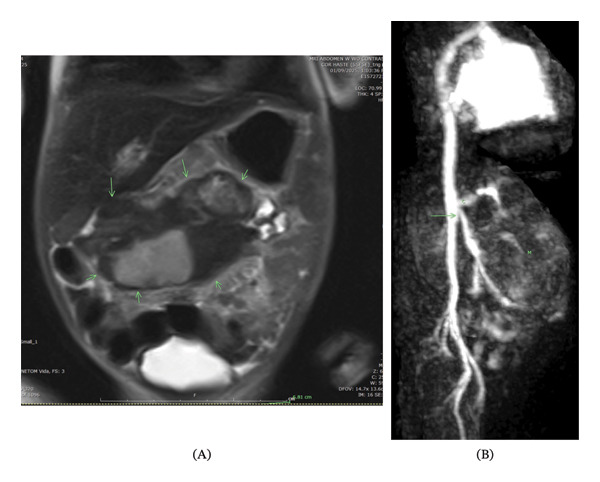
(A) Coronal T2‐weighted MRI images demonstrate a heterogeneous mass lesion centered in the mesentery, which corresponds to the ultrasound findings. (B) MRA images demonstrate that the mass (M) is perfused by the superior mesenteric artery (arrow) and the celiac axis (C).
